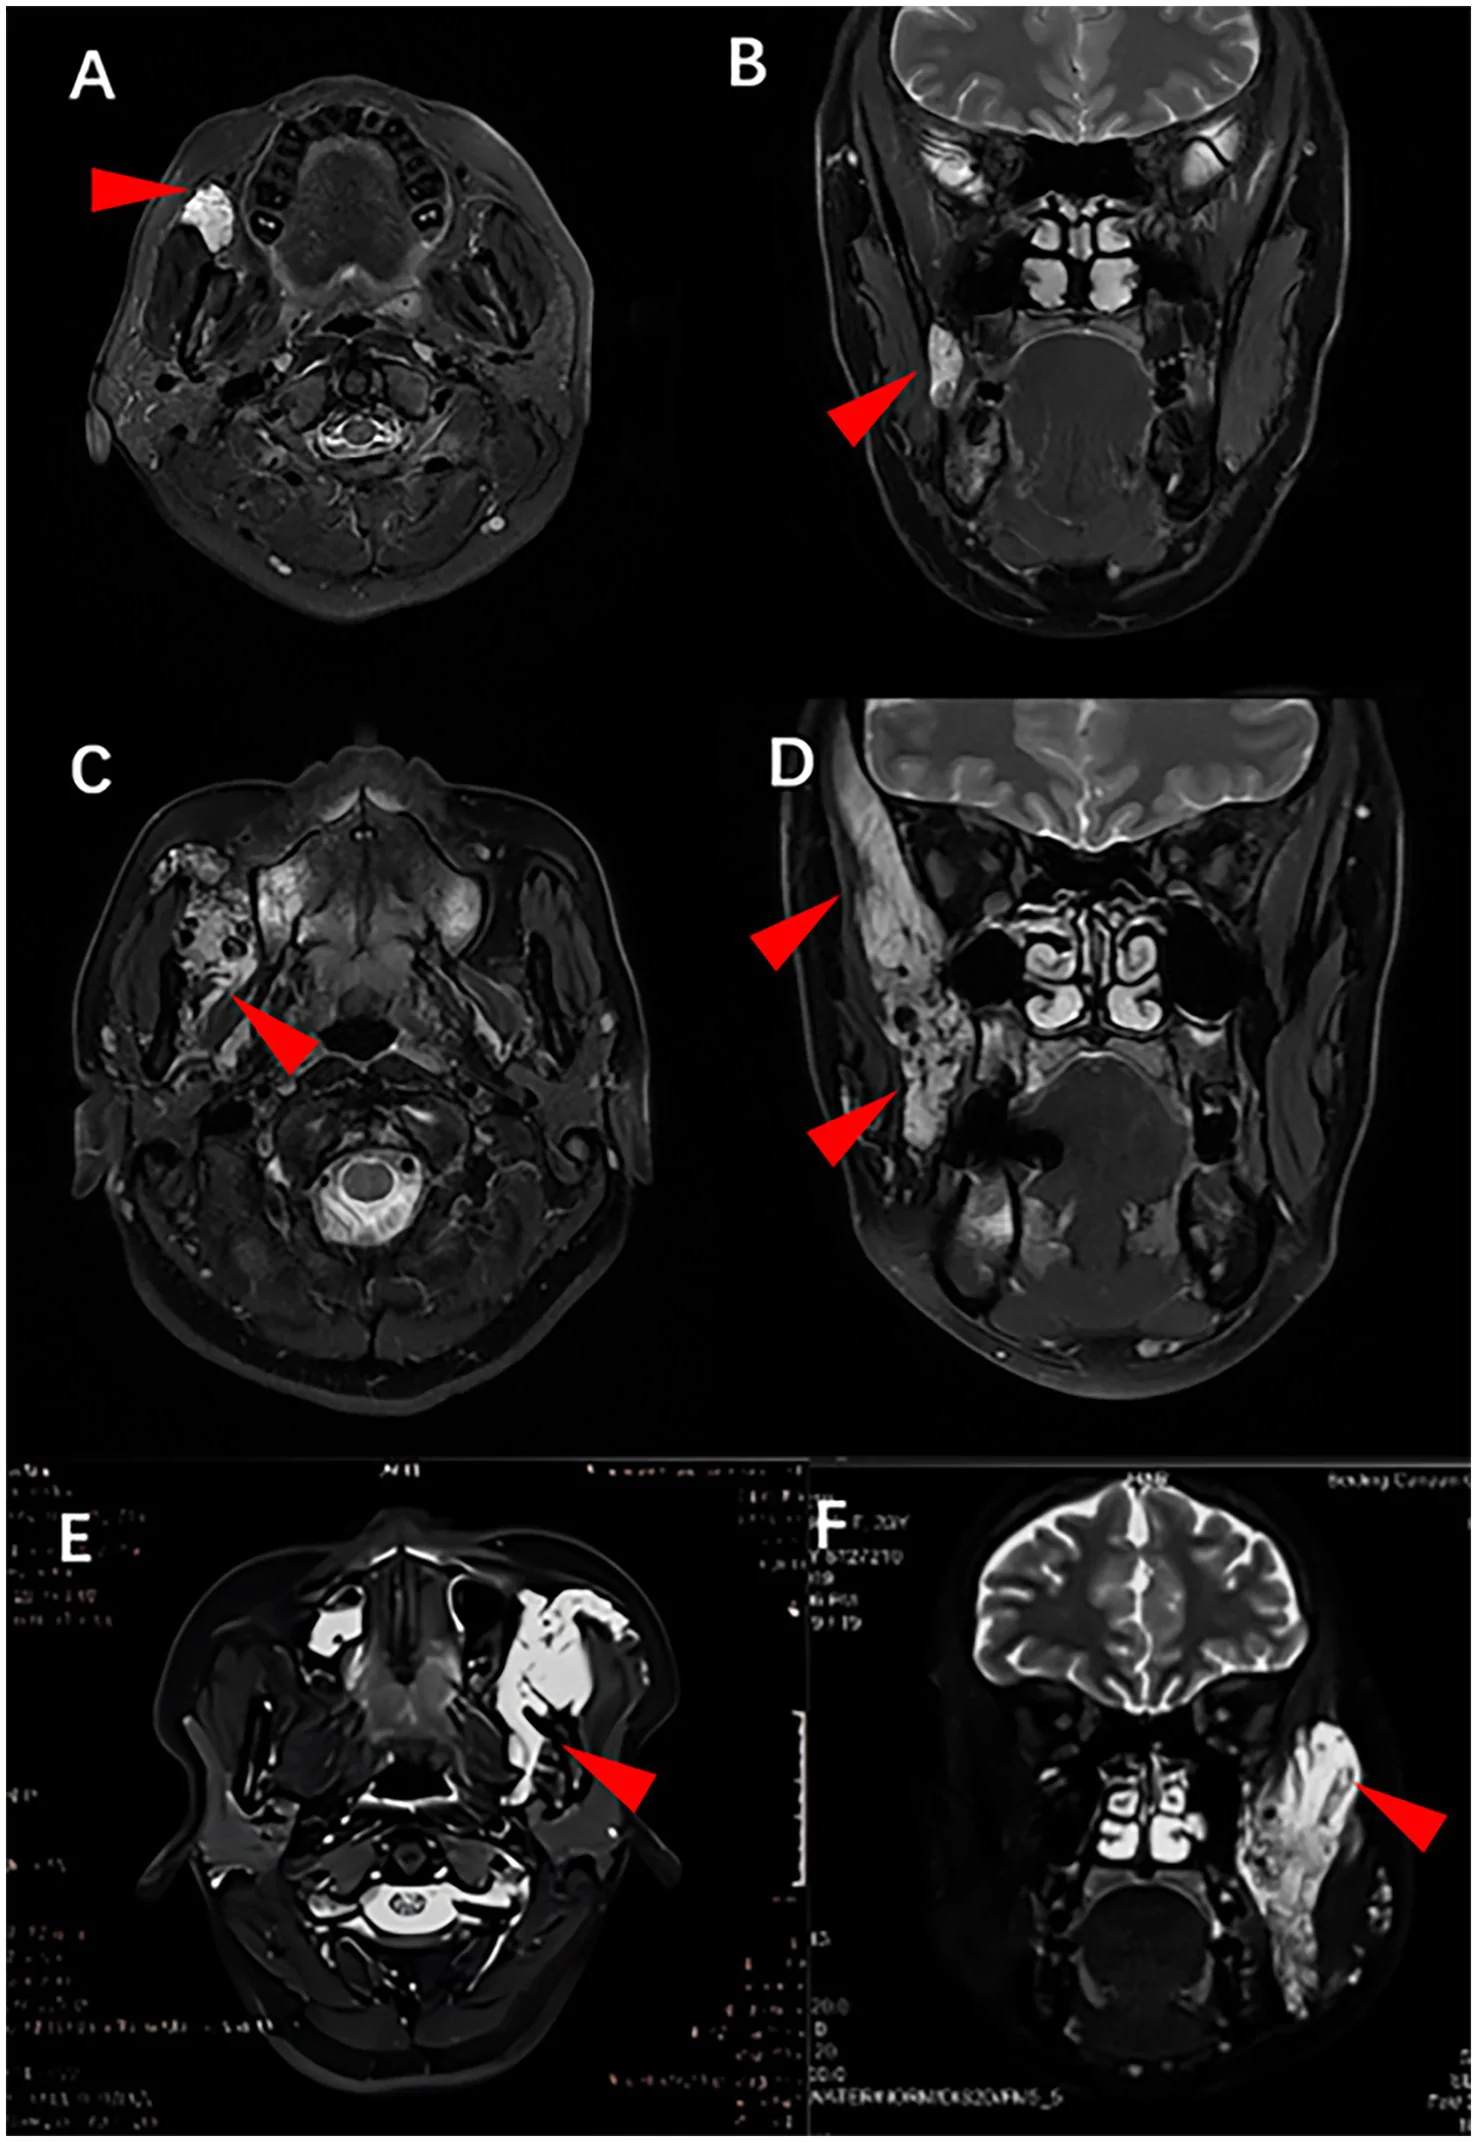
Representative MRI findings of the extension-based classification of buccal fat pad venous malformations. (A,B) Type I lesion confined predominantly to the body and buccal component of the buccal fat pad. (C,D) Type II lesion extending beyond the buccal component into adjacent deep facial spaces. (E,F) Type III lesion with extensive deep-space involvement and clinically significant zygomatic asymmetry.
